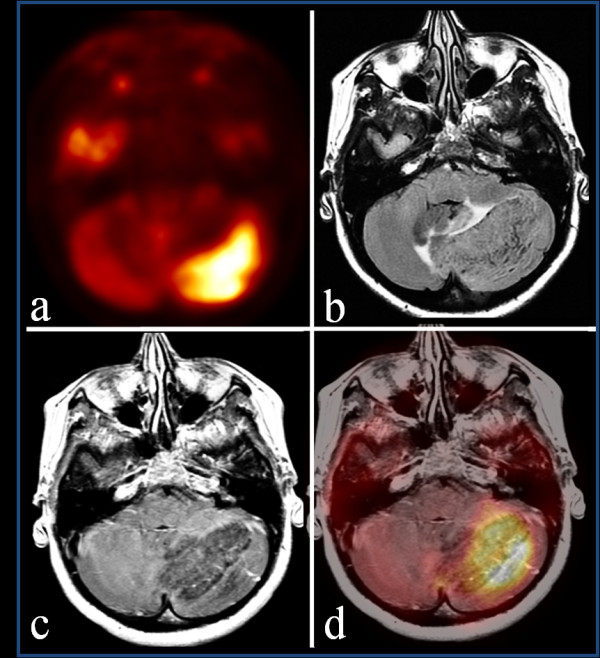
Axial positron emission tomography view. (a) Intense glucose metabolism in the left hemisphere of the cerebellum, corresponding to a hypointense and slightly enhancing area with (b) maximum diameter of 5 cm in axial T1-weighted post contrast view. (c) Axial T2-weighted fluid-attenuated inversion recovery images show the characteristic features of Lhermitte-Duclos disease: striations representing the folia of the cerebellum in association with hydrocephalus and dislocation of the fourth ventricle. (d) Axial fused positron emission tomography-magnetic resonance imaging views show the lesion with increased metabolism and intensity signal similar to that of normal grey matter.
